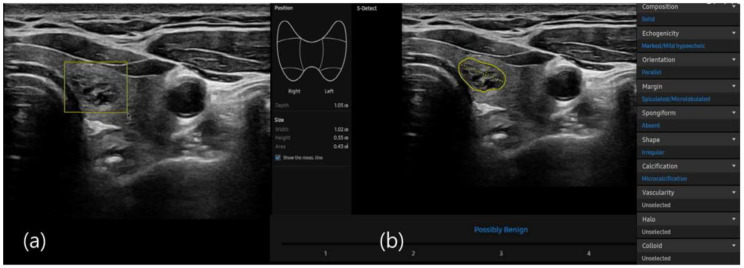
(a) Ultrasound grayscale transverse image of the left thyroid lobe in a 57-year-old woman. (b) Thyroid nodules automatically classified as “possibly benign” using S-detect according to characteristics such as internal composition, echogenicity, orientation, margin, and shape.
